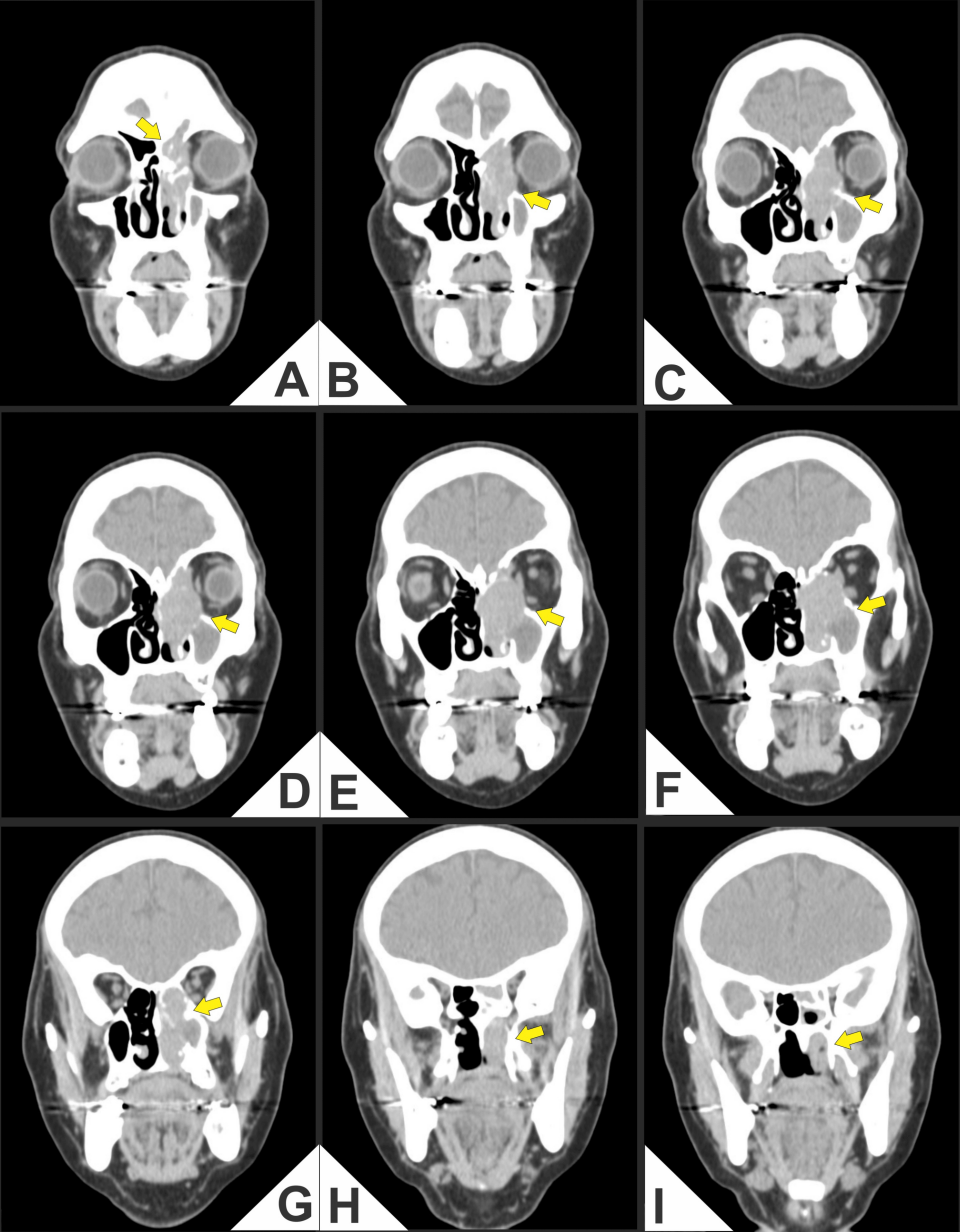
(A-I) Coronal computed tomography (CT; soft-tissue window throughout) of the paranasal sinuses and anterior skull base. Heterogeneously enhancing soft-tissue mass centered in the left nasoethmoid-maxillary complex (arrows). (A) Cortical irregularity and a focal defect of the fovea ethmoidalis/cribriform plate, suggesting anterior skull base involvement. (B, C) Tumor filling the left anterior ethmoid air cells with dehiscence of the lamina papyracea and medial extraconal orbital extension, characterized by contact and flattening of the medial rectus muscle and effacement of the extraconal fat plane, without definite intraconal invasion. (D-F) Persistent heterogeneous enhancement with internal hypodense areas and continued erosion of the medial orbital wall; the globe contour and attenuation are preserved. (G-I) Near-complete opacification of the left maxillary sinus with remodeling/erosion of its medial wall and subtle fullness/stranding in the left pterygopalatine fossa, suggesting early posterior extension.
